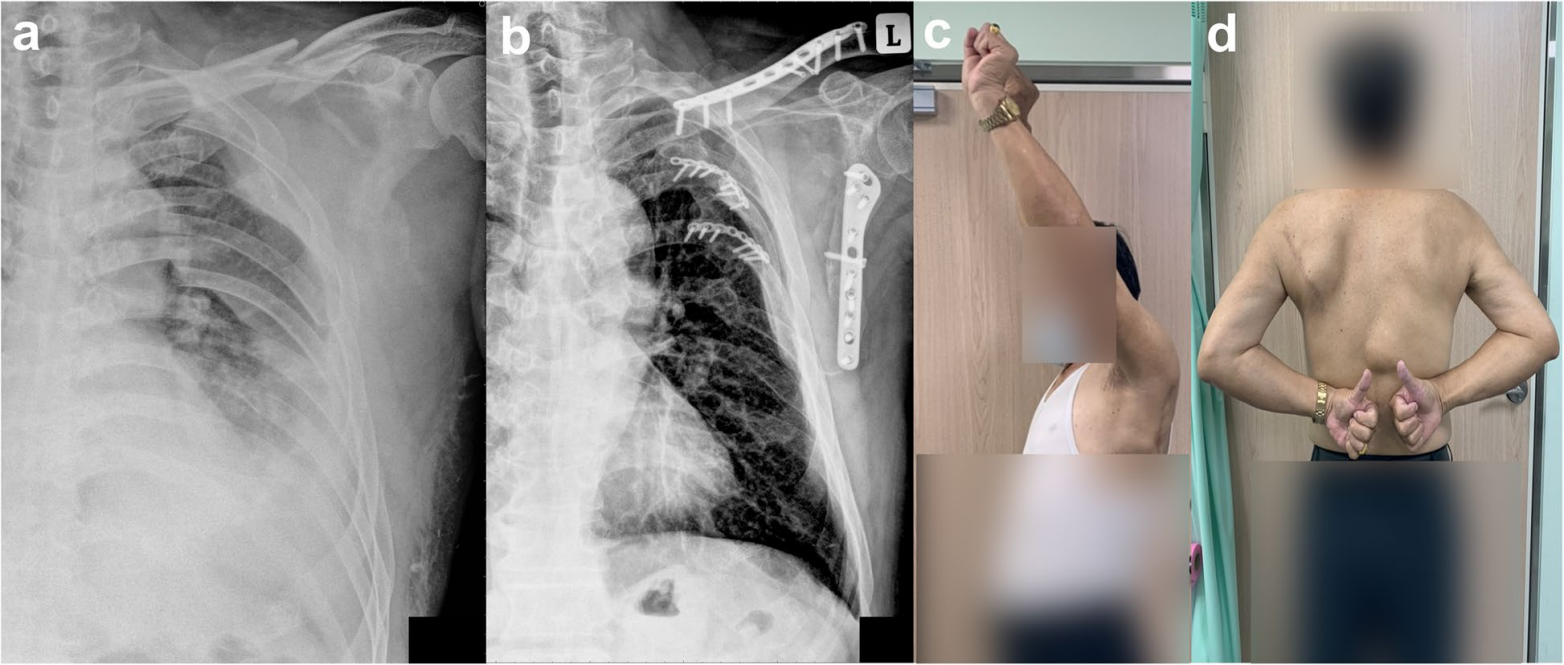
A 60-year-old male (case No. 2) presented a left scapula fracture (AO/OTA 14B(lm) type), ipsilateral clavicle and second to seventh rib fractures with hemothorax and pneumothorax (a). Using the mirror Judet approach, the scapula and the fourth and fifth ribs were reduced and stabilized. At the 12-month follow-up, the radiograph showed union of both the scapula and rib fractures (b). He had already returned to his original work with a shoulder ROM comparable with that of the other side (c, d) and three points in the Disabilities of Arm, Shoulder, and Hand score
